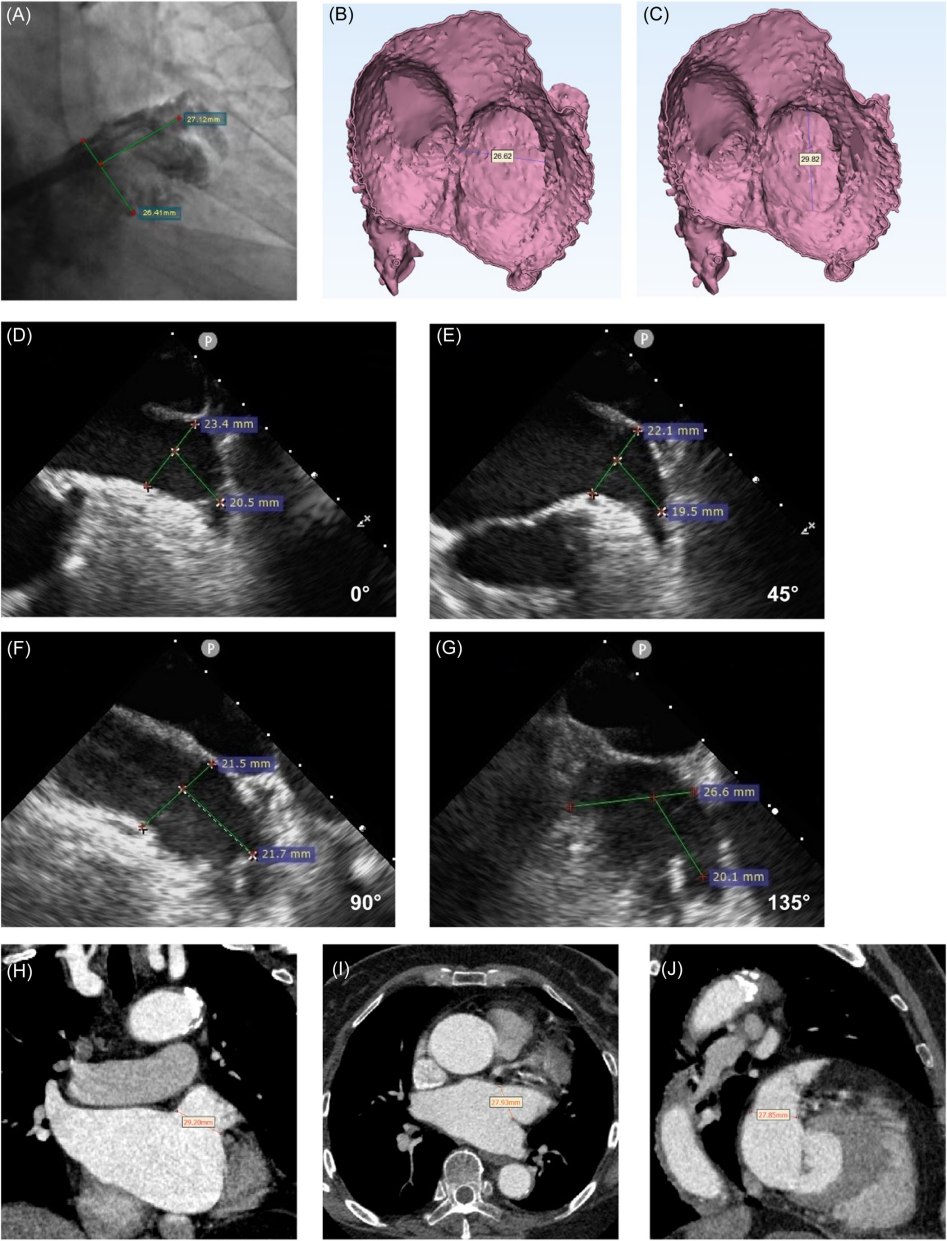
LAA orifice diameter as measured by different imaging methods. (A) Digital subtraction angiography at the 30° right anterior oblique position +20° caudal position to measure the LAA orifice diameter. (B, C) LAA orifice diameter was measured by 3D reconstruction of CTA using Mimics software. (D–G) LAA orifice diameter was measured in four sections at 0°, 45°, 90°, and 135°, respectively, by transesophageal echocardiography. (H–J) LAA orifice diameter was measured by multiplanar reconstruction with CT angiography in different positions (coronal, sagittal, and transverse positions). CT, computed tomography; CTA, computed tomography angiography; LAA, left atrial appendage.
